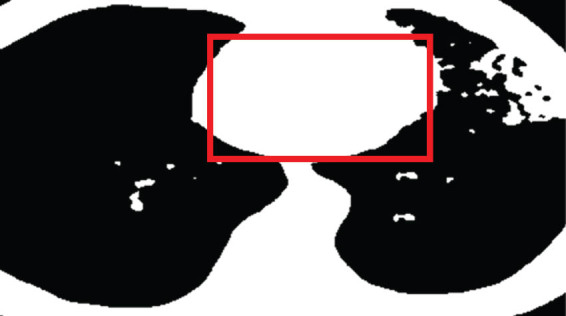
Patient CT image (Red box indicates ROI). The patient was a 55-year-old male with intermittent cough and wheezing for 5 years, aggravating phlegm with blood and high fever for 11 days. Ten days before admission, the patient had no obvious cause of phlegm and blood, and pain in the left chest area (initial examination on admission).
